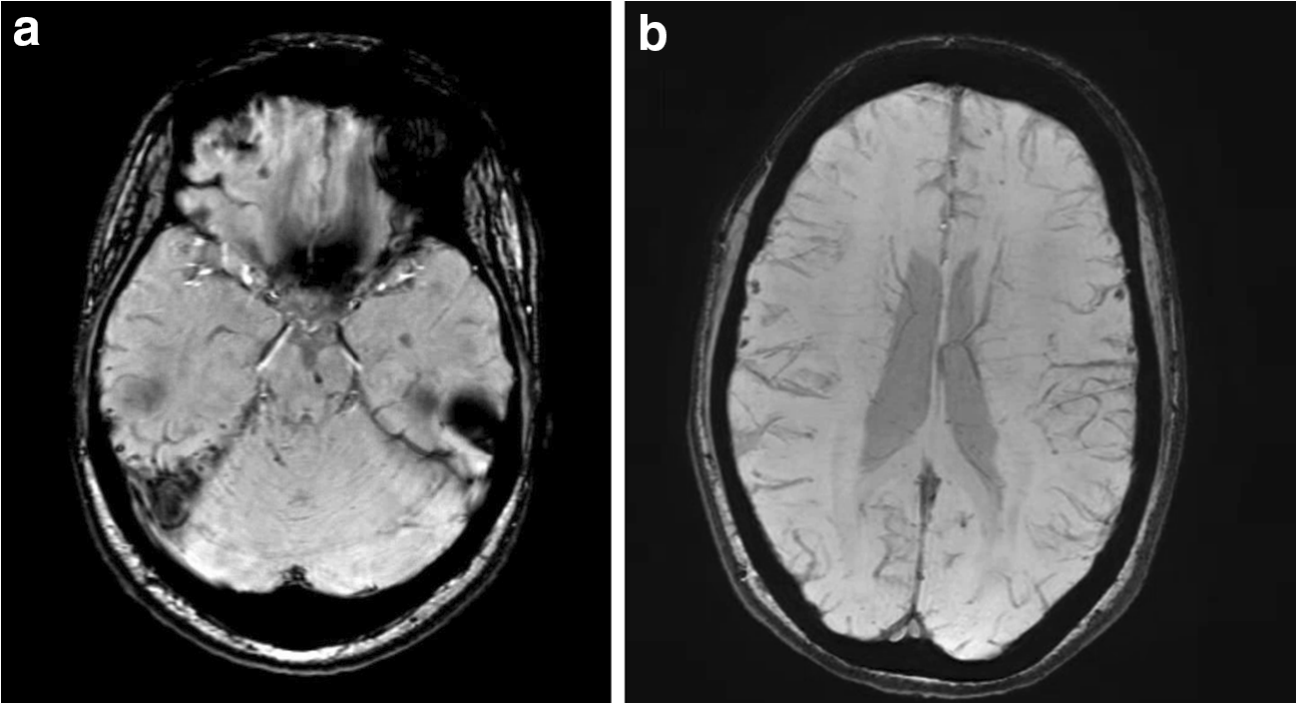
Susceptibility-weighted imaging (SWI) MRI series of a young patient with TAI. Note the susceptibility artifacts in the brain stem, temporal lobes, and cerebellum (a) and splenium of the corpus callosum (b). Despite grade 3 TAI, the patient was awake and communicating
